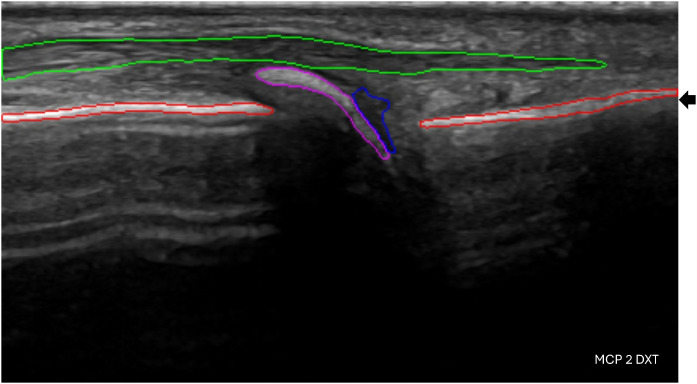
Example output from the AI segmentation and disease activity scoring model (DIANA). The image shows a longitudinal dorsal view of a metacarpophalangeal (MCP) joint. Bone is marked in red, tendon in green, synovium and cartilage combined in blue, and bone with osteophyte formation in purple. The ultrasound machine’s focus point is marked using an arrow.
